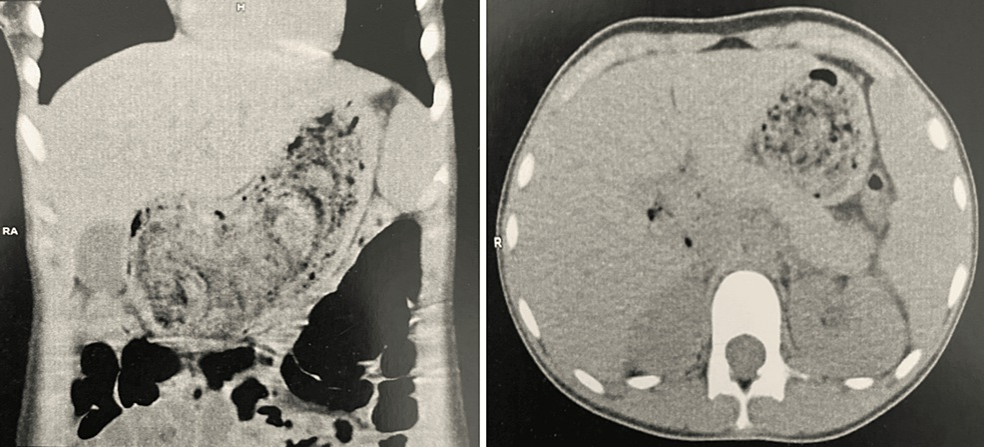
Abdominal CT scan with frontal (left) and axial (right) section showing the presence of an intragastric foreign body, with entrapped air within its tangles, suggesting a trichobezoar.
